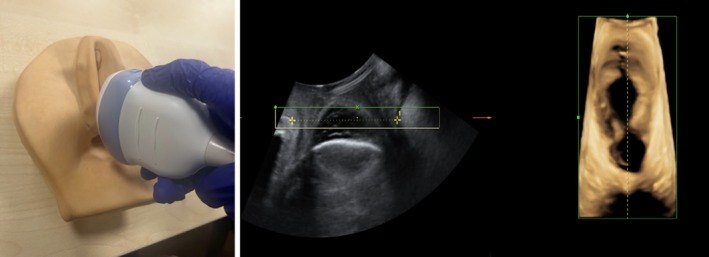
Transperineal 3D ultrasound in the midsagittal plane with corresponding 2D and 3D render image at the plane of minimal hiatal dimension.
